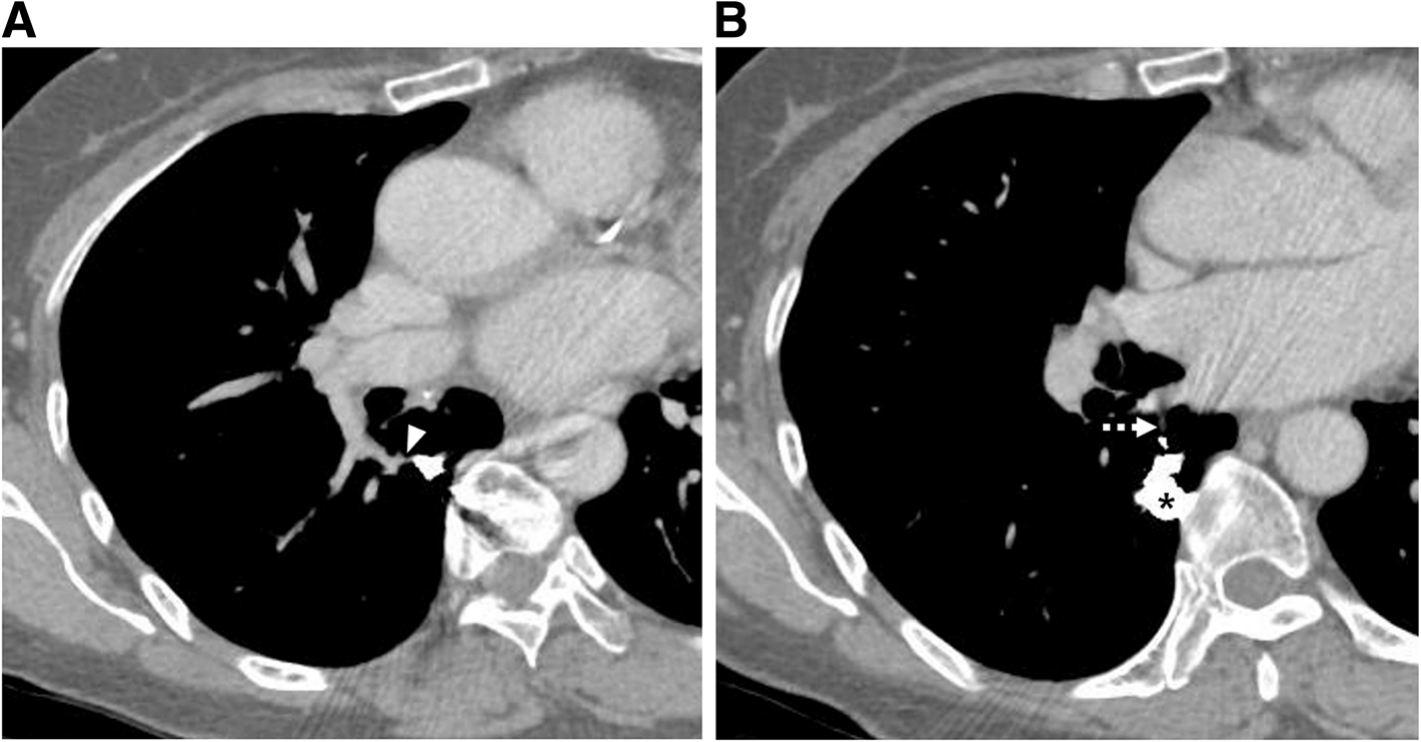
A, B After five months of PAVM embolization, the venous sac packed with coil nest (asterisk) and a significant reduction in the size of the feeding artery (arrowhead) and draining vein (dashed arrow) are observed on reconstructed CT images
